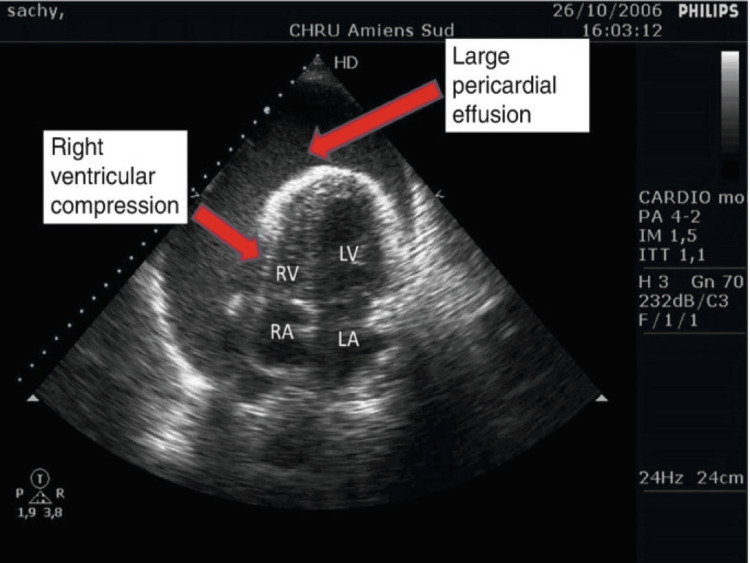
Example of large pericardial effusion and RV compression on TEE via a deep transgastric five-chamber view RV: right ventricular; TEE: transesophageal echocardiography
